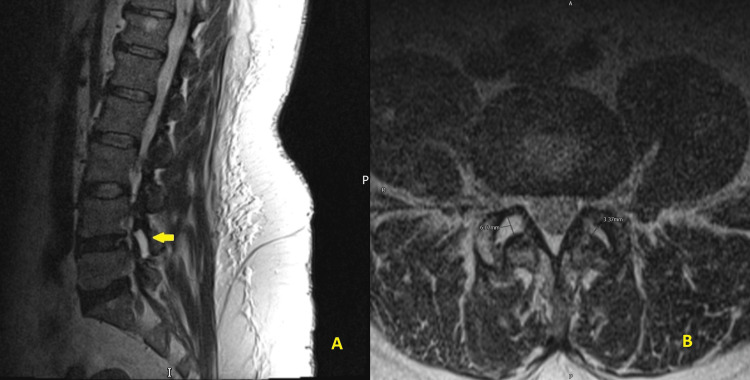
(A) T2W right para sagittal MRI LS spine showing right facet joint fluid (yellow arrow). (B) T2W axial MRI LS spine at L4-L5 intervertebral disc level showing bilateral facet joint fluid with a depth of 6 mm on the right and 3.3 mm on the left side LS spine, lumbosacral spine; T2W, T2-weighted
